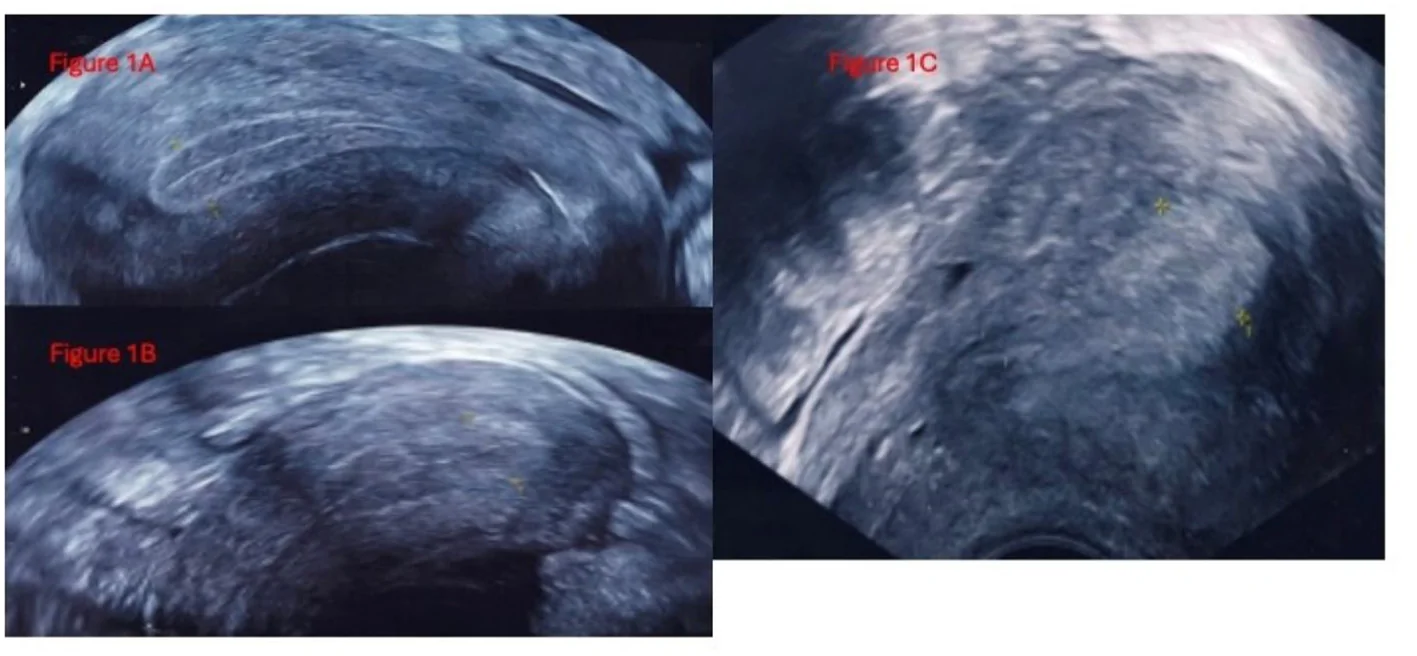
Transvaginal ultrasound images of endometrial pattern types. (A) Type A: A clear three-layered “triple-line” endometrium consisting of a central hyperechoic line within a homogeneous endometrium bounded by two hyperechoic peripheral layers. (B) Type B: A partially trilaminar but less distinct endometrium with an irregular or poorly defined central and/or outer line. (C) Type C: A uniformly hyperechoic endometrium without a visible triple-line structure.
